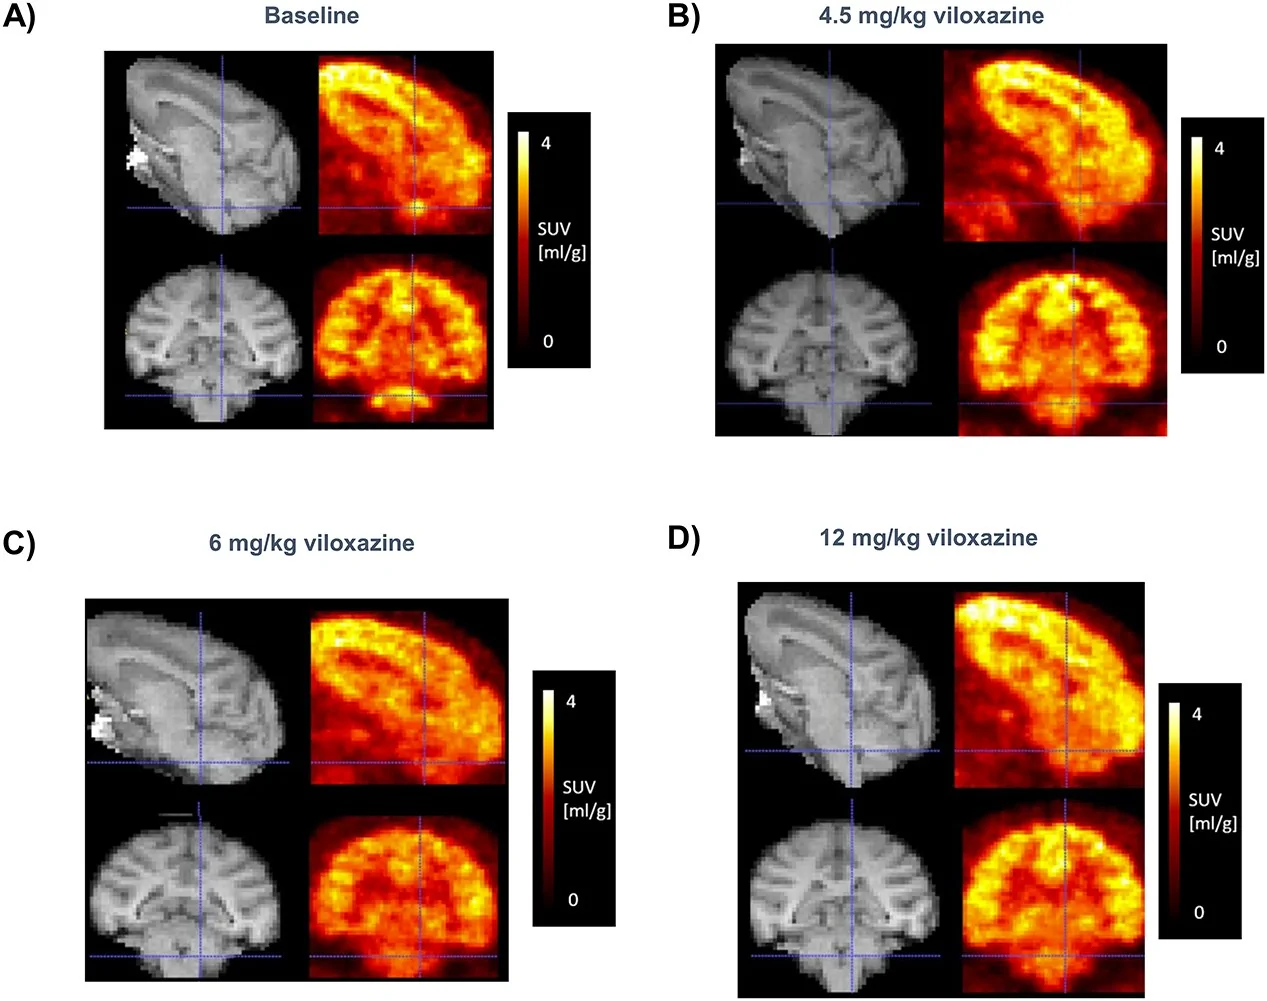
Representative [^11^C]CIMBI-36 CT and PET images of NHP001 showing expected radioligand uptake at (A) baseline, and after administration of (B) 4.5, (C) 6, and (D) 12 mg/kg of viloxazine scaled to SUVs along with an MRI template of the brain. Abbreviations: CT = computed tomography; PET = positron emission tomography; SUV = standardized uptake value.
